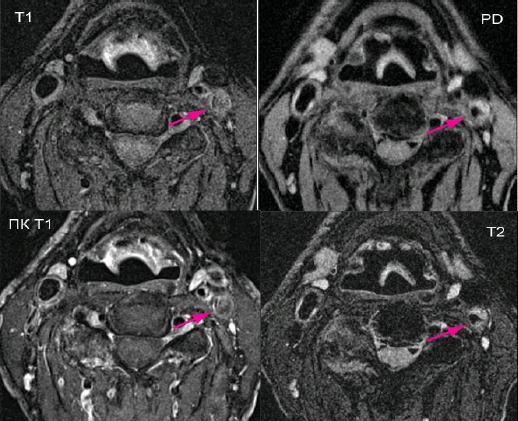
MRI of unstable carotid plaque in 67 years old symptomatic male, with ulceration (type VIa), occluding 95% of the lumen of the left ICA. It consists of a large lipid core, plaque hemorrhage and a thin fibrous cap (enhanced on postcontrast T1), in which a small area is interrupted (ulceration)*.
